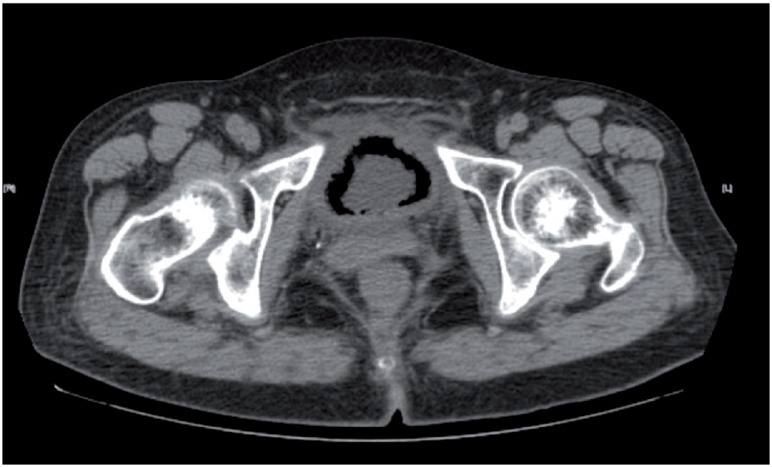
Gas surround the bladder wall on computed tomography.
